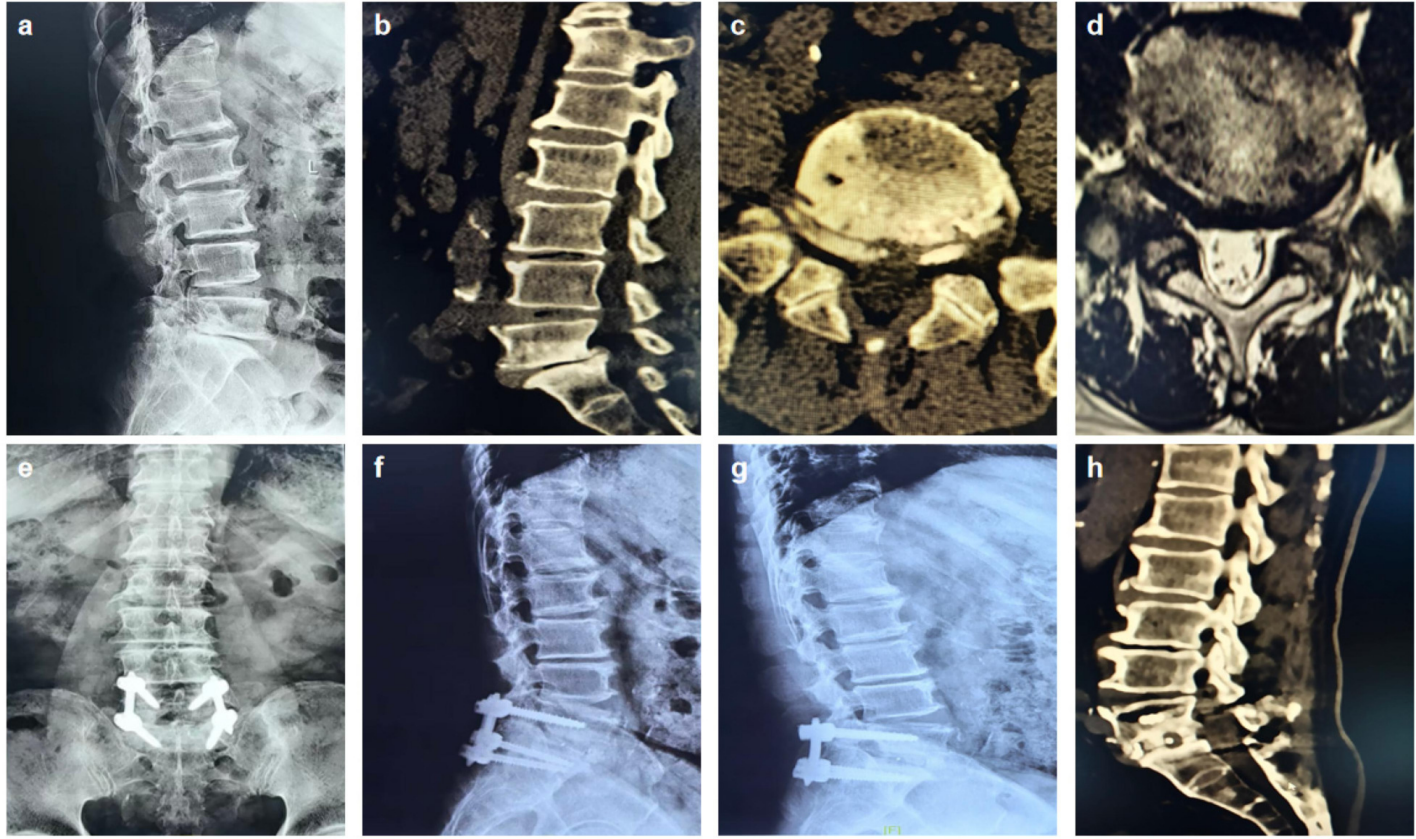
Imaging data of a typical case in Endo-PLIF A 72-year-old male patient. Preoperative DR, CT and MRI show grade I lumbar spondylolisthesis (a,b,c,d). Postoperative 3-month X-rays show well-positioned pedicle screws and interbody cage (e,f). Postoperative 12-month X-ray demonstrates maintained satisfactory positional stability of the cage and implants (g). Postoperative 12-month CT scans confirm satisfactory positioning of the implants and solid bony interbody fusion at Bridwell Grade I (h).
